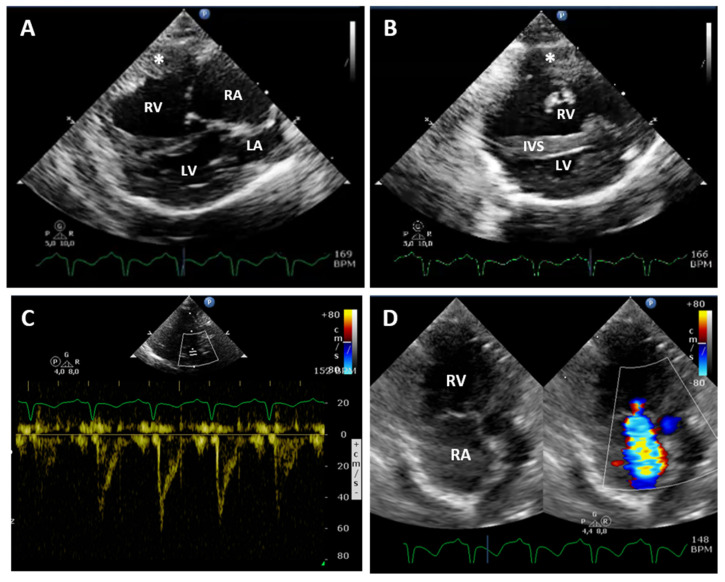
Echocardiography at presentation: (A,B) Right parasternal window long-axis 4-chamber view and short-axis view at the level of papillary muscles, respectively. Severe right atrium and ventricle dilation, right ventricle free wall hypertrophy and a reduced left ventricular diameter were evident. (C) Pulsed-wave Doppler tracing of the pulmonary artery systolic flow: the laminar flow profile is asymmetrical with a sharp peak, corresponding to type II pulmonary blood flow. (D) Left parasternal window apical view optimized for the right heart. On color flow mapping (on the right), moderate-severe tricuspid regurgitation is evident. RA, right atrium; RV, right ventricle; IVS, interventricular septum; LA, left atrium; LV, left ventricle; *, right ventricular free wall.
